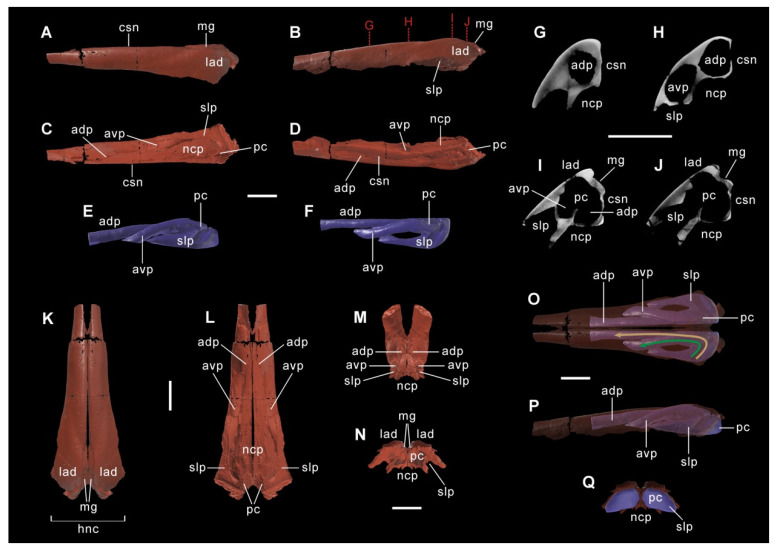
Images on 3D reconstruction of the main body of the left nasal and its accessory internal cavity by CT scans. 3D model of the main body of the left nasal in dorsal (A), lateral (B), ventral (C) and medial (D) views. 3D model of the left accessory endonasal cavity in lateral (E) and dorsal (F) views. CT cross sections along the posterior half of the left nasal in anteroposterior sequence (G–J). 3D model of the combined main body of the left nasal and its mirror copy in dorsal (K), ventral (L), anteroventral (M) and posterior (N) views. Translucent 3D model of the combined main body of the left nasal and its mirror copy showing the paired accessory endonasal cavities in dorsal (O), left lateral (P) and posterior (Q) views. The airways via the anterodorsal and anteroventral passages are indicated by the golden and green arrows, respectively. Scale bar equals 10 cm.
